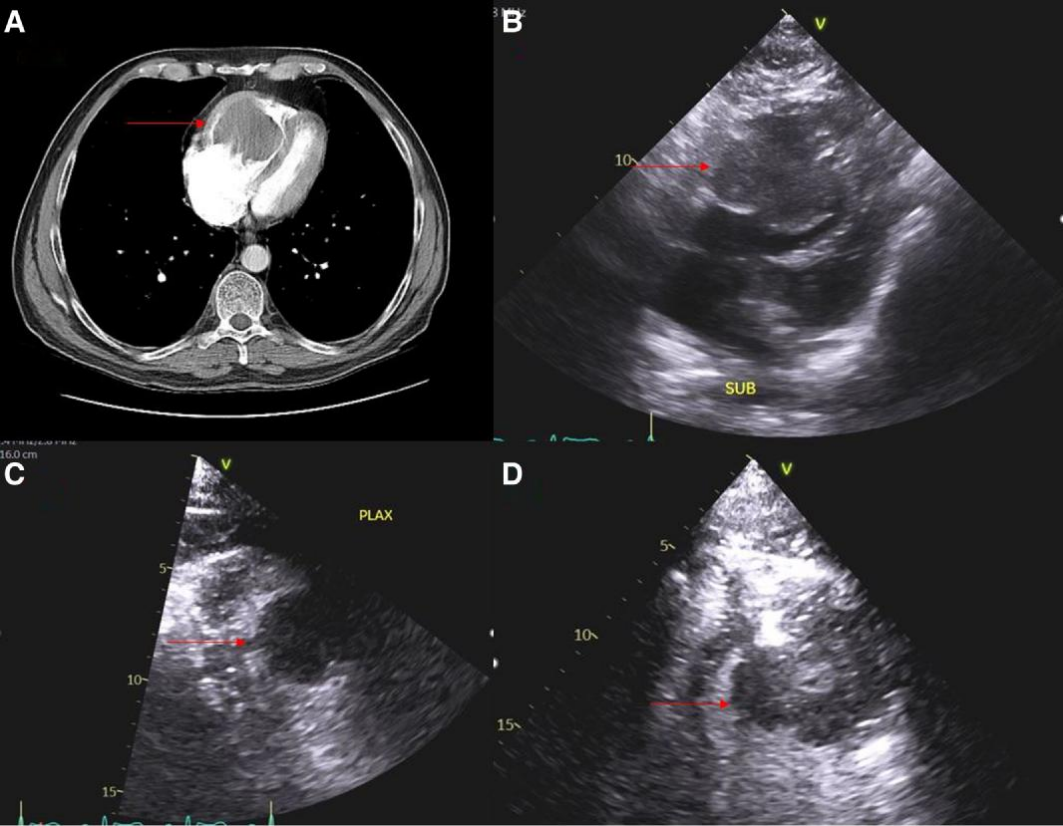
CT and echocardiographic images. (*A*) CT pulmonary angiogram axial view demonstrating large right ventricular mass (arrow) with septal displacement. (*B*) Transthoracic echocardiogram subcostal view corresponding to CT image demonstrating right ventricular mass (arrow) compressing left ventricle. (*C*) Transthoracic echocardiogram parasternal long axis view with imaging enhancer showing right ventricular mass. (*D*) Transthoracic echocardiogram apical four-chamber view with imaging enhancer demonstrating right ventricular mass (arrow).
